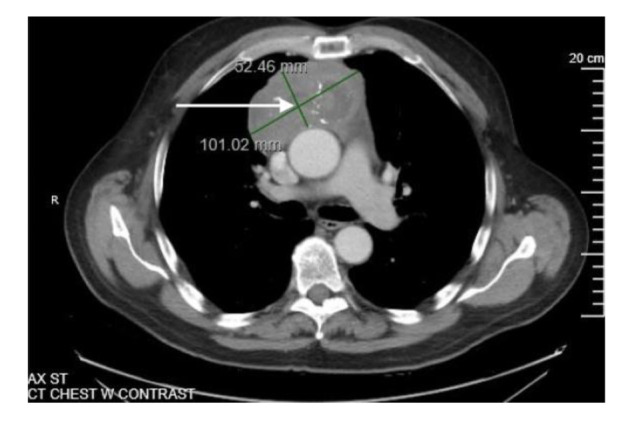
Computed tomography for the thoracic region findings of conglomerate soft tissue and a heterogeneously dense mass with calcifications and cystic components in the anterior mediastinum measuring 10.1 x 5.2 cm extending into the substernal space measuring 10.7 cm superior to inferior
